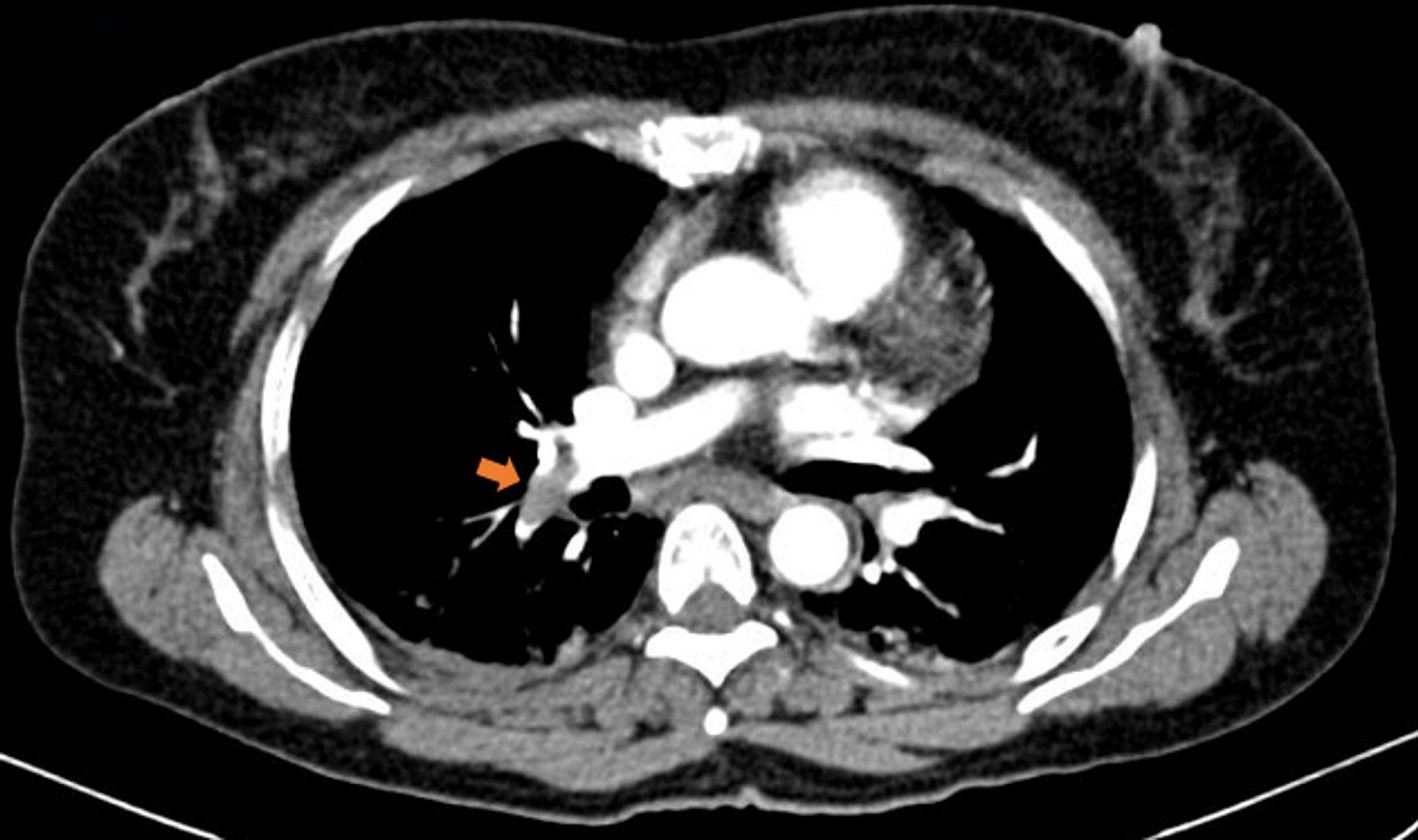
Axial-section image of the contrast-enhanced computed tomography (CECT) of thorax showing a hypodense filling defect (arrow) in the inferior branch of right pulmonary artery suggestive of acute pulmonary thromboembolism.
